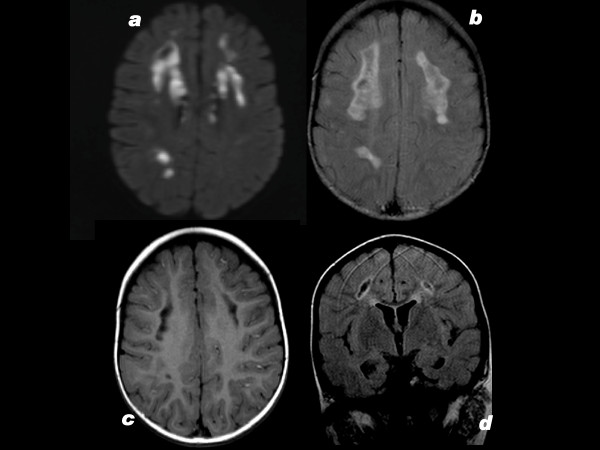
Patient 1, brain MRI. 1a: TRACE sequence shows cytotoxic oedema in frontoparietal white matter bilaterally, and in right occipital. 1b: FLAIR sequence, right occipital and bilateral frontoparietal lesions; compare to fig 1a. 1c: 5 months later. T1 weighted without iv contrast. Bifrontal white matter loss with lacunae, small lacuna in right occipital. 1d: Coronal section; bilateral loss of white matter; lacunae lined by high signal interpreted as gliosis.
